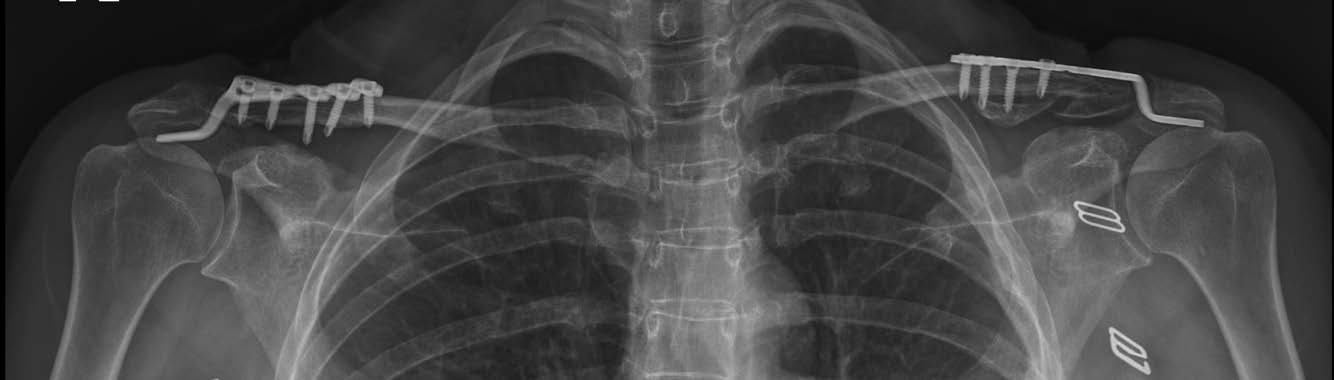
Postoperative late-period radiography (fifth year).
